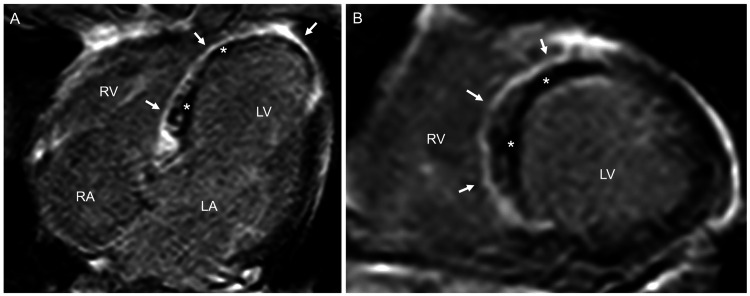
Delayed contrast-enhanced cardiac magnetic resonance images (A, four-chamber view; B, short-axis view) obtained on the ninth hospital day Images show a transmural hyperenhancement (arrows) in the whole area at risk of a myocardial infarction, which corresponded to the necrotic area and surrounded a dark core (asterisks) that reflected the microvascular obstruction. LA, left atrium; LV, left ventricle; RV, right ventricle; RA, right atrium
